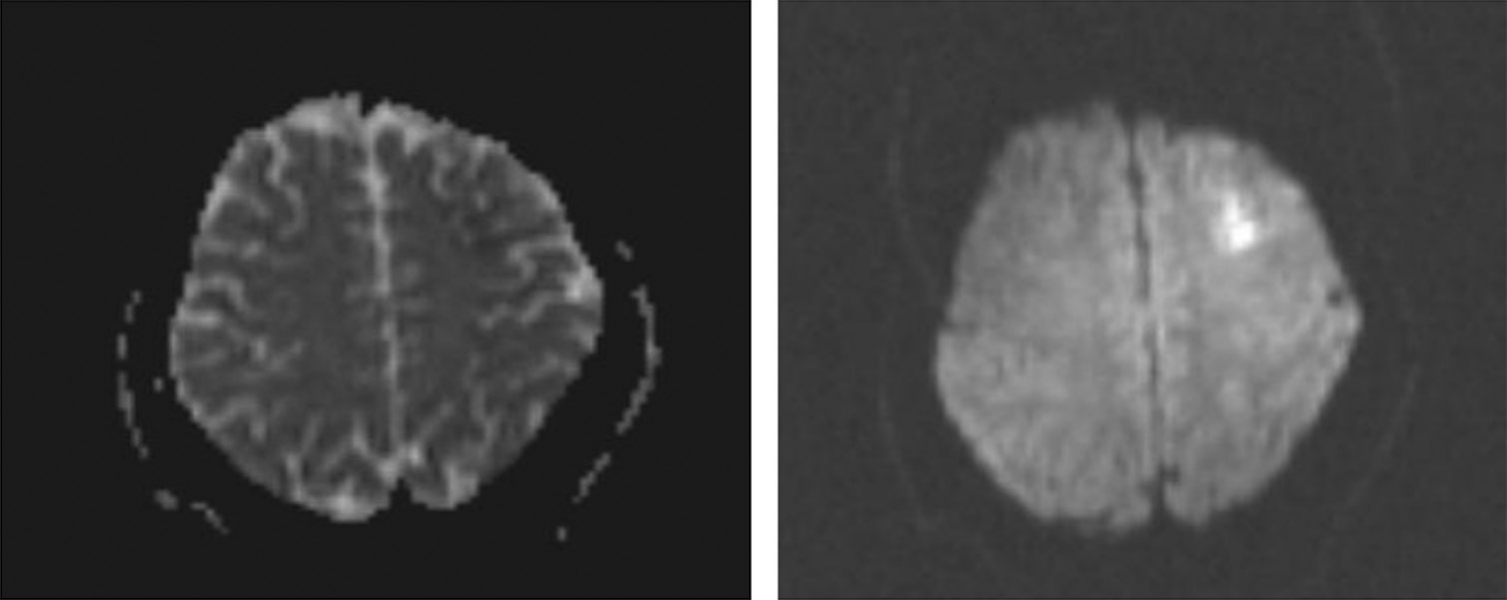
Brain MRI revealed abnormal signal intensity in the left frontal region in T2/FLAIR, which shows restriction in DWI image in favor of acute infarction in left MCA territory
